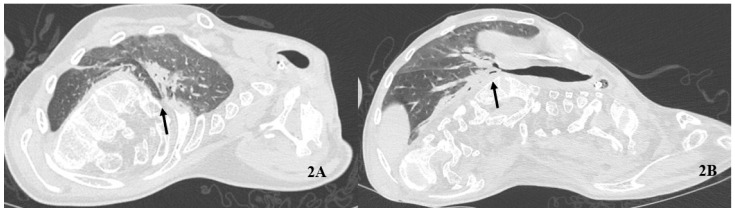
Follow-up computed tomography of the chest. The arrows indicate the sub-total resolution of consolidations at hilum (A) and the right lower lobe (B).
